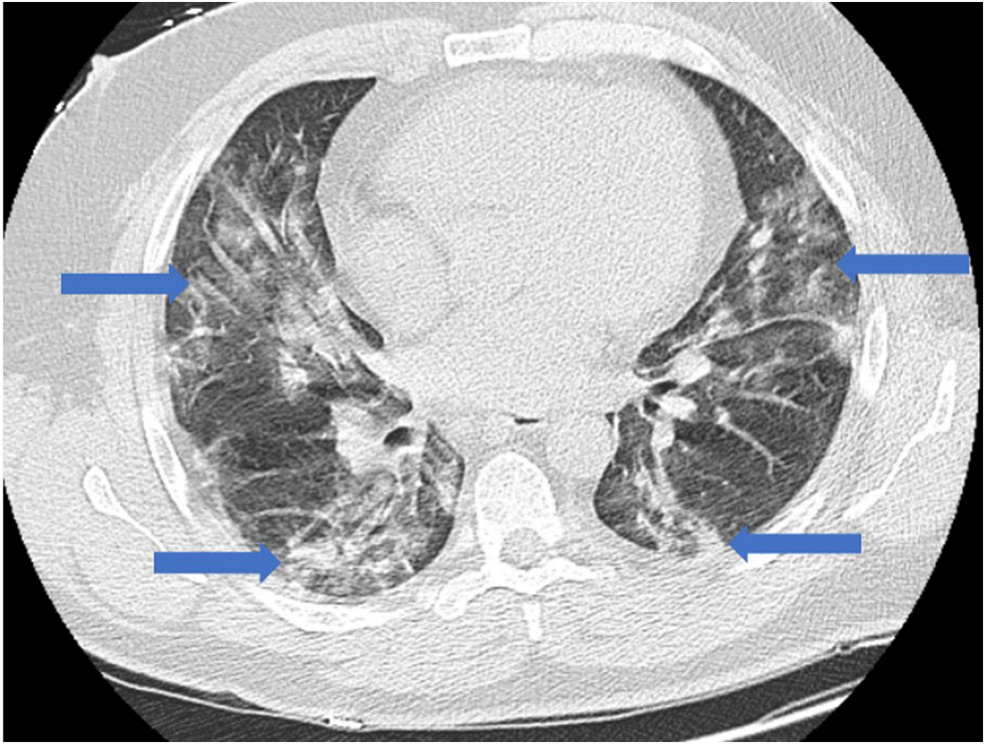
Early stage COVID-19 (0–4 days): Axial CT scan of the chest in a 52-year-old man with COVID-19 predominantly demonstrates peripheral ground glass opacities (blue arrows).
